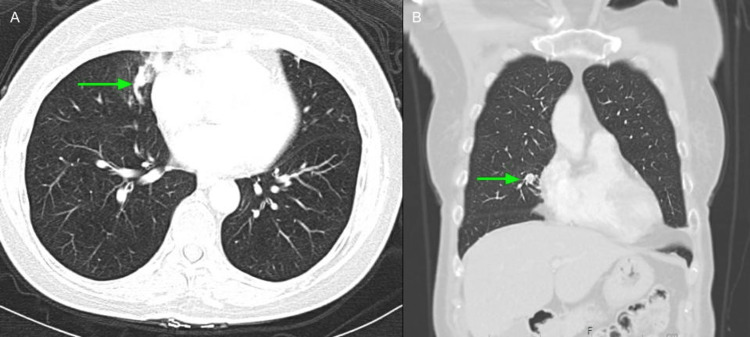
Chest CT at 11 months showed an interval increase in the right middle lobe nodule (green arrow) concerning tumor progression (A: axial view, B: coronal view).
